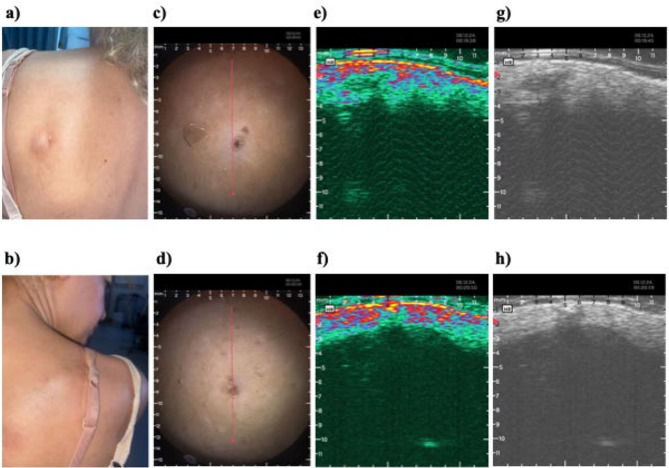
Epidermoid cysts in a 55-year-old female patient, Fitzpatrick skin type II. a, b Clinical images showing skin-coloured, round, regularly shaped, raised lesions on the left scapular region, slightly lighter than the surrounding skin, with a small central punctum. c, d Dermoscopic image of the lesions. e, g Dermoscopy-guided high-frequency ultrasound (DG-HFUS) images of lesion 1, demonstrating a dermal lesion with a depth of 2.1 mm. f, h DG-HFUS images of lesion 2, showing a dermal lesion with a depth of 1.4 mm. Both lesions appear less hyperechoic than the surrounding dermis, with focal hyperechoic areas and irregular, star-like protrusions extending into the adjacent tissue, likely corresponding to collagen bundles linking the cysts to neighbouring dermal structures. In both cases the submarine sign can be observed
